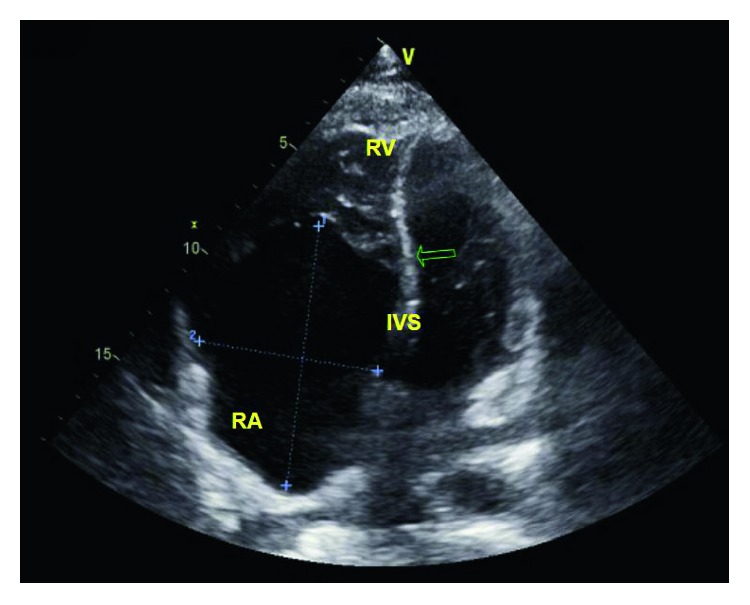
Apical 4 view showing apical displacement of the tricuspid valve leaflet causing atrialization of the right ventricle. This is diagnostic of Ebstein's anomaly.
